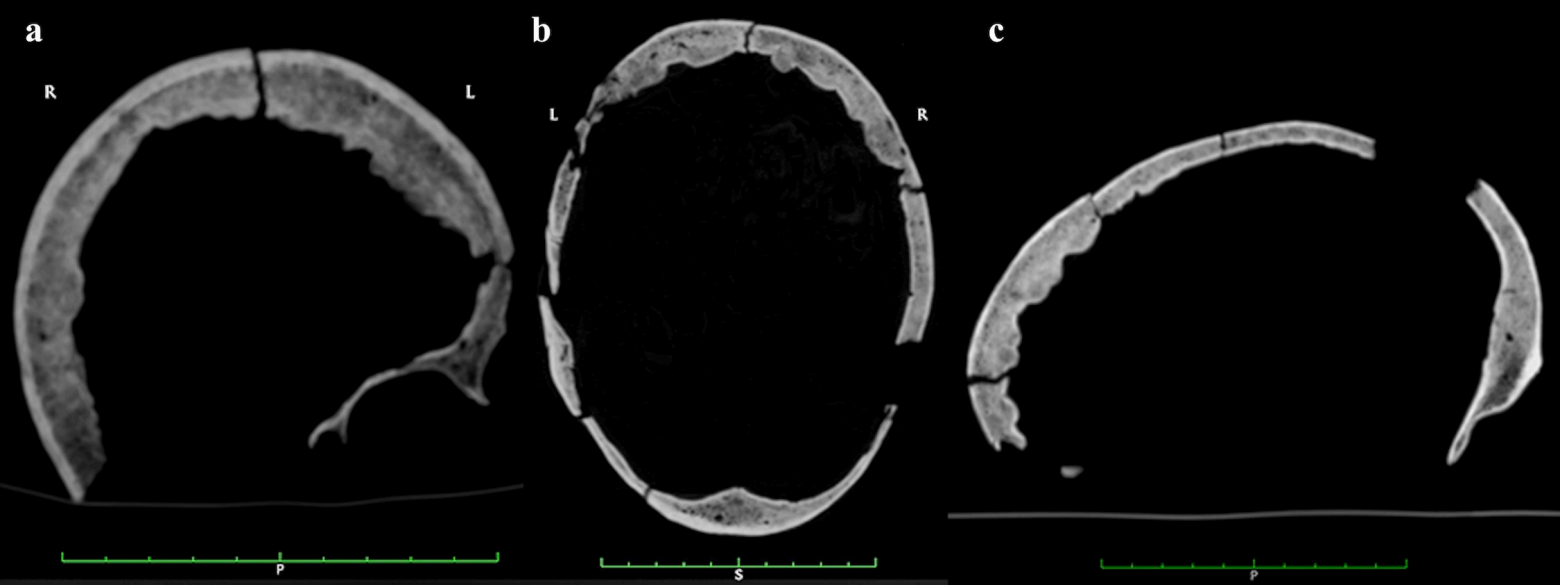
CT-scans in all projections: a. coronal view, b. axial view, c. sagittal view. The thickening and extent of the bony growth in the form of nodules protruding into the cranial cavity can be appreciated. A general thickening of the parietal and occipital bones can also be seen.
